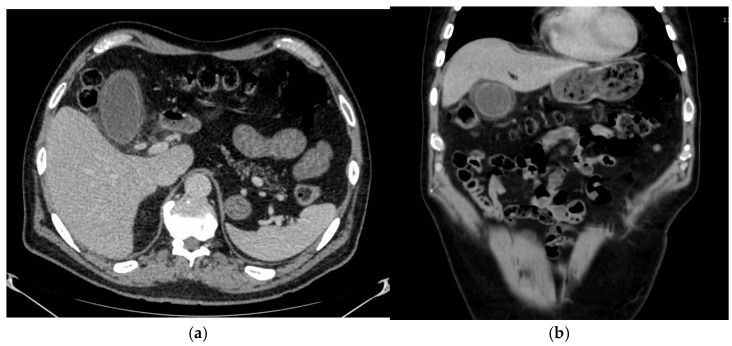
(a) horizontal plane view of a CT of abdomen with contrast: increased enhancement of the gallbladder wall with pericholecystic edema and fat stranding extending to common bile duct (b) transverse plane view of a CT of abdomen with contrast: increased enhancement of the gallbladder wall with pericholecystic edema and fat stranding extending to common bile duct and first part of duodenum.
